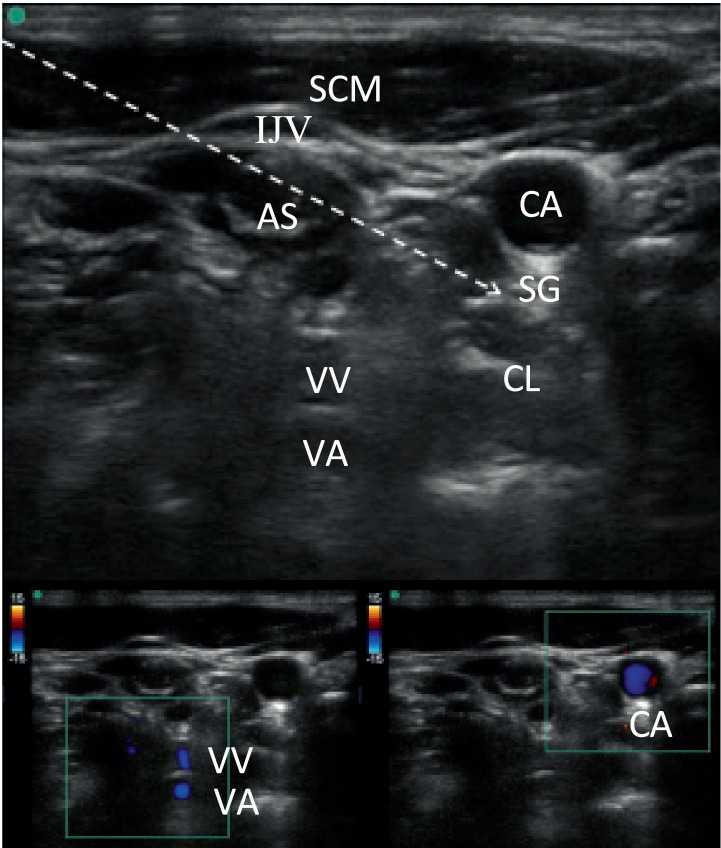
Ultrasound-guided stellate ganglion block. SCM, sternocleidomastoid muscle; IJV, internal jugular vein; CA, carotid artery; AS, anterior scalene; VV, vertebral vein; VA, vertebral artery; SG, stellate ganglion; LCM, longus colli muscle.
